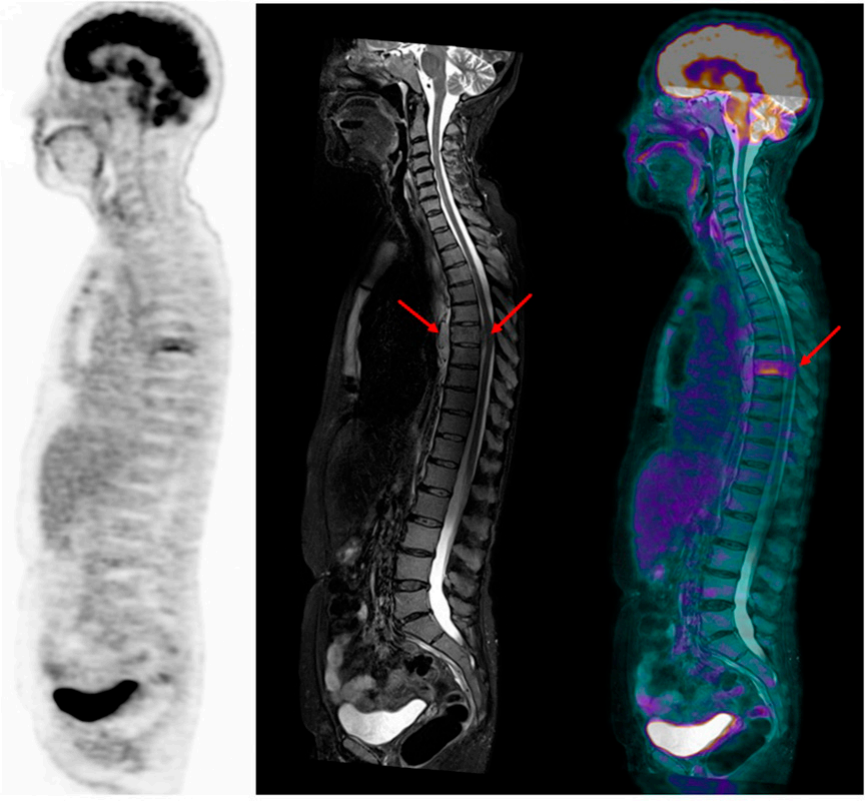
Sagittal PET (left), T2-weighted short-τ inversion recovery MR (middle), and PET/MR (right) images. Homogeneous diffuse bone marrow involvement is seen on MRI (diffuse homogeneous increased signal), with added FL in T7 body (with ^18^F-FDG avidity). Anterior and posterior soft-tissue extension (arrows) defines paramedullary disease and leads to cord compression.
